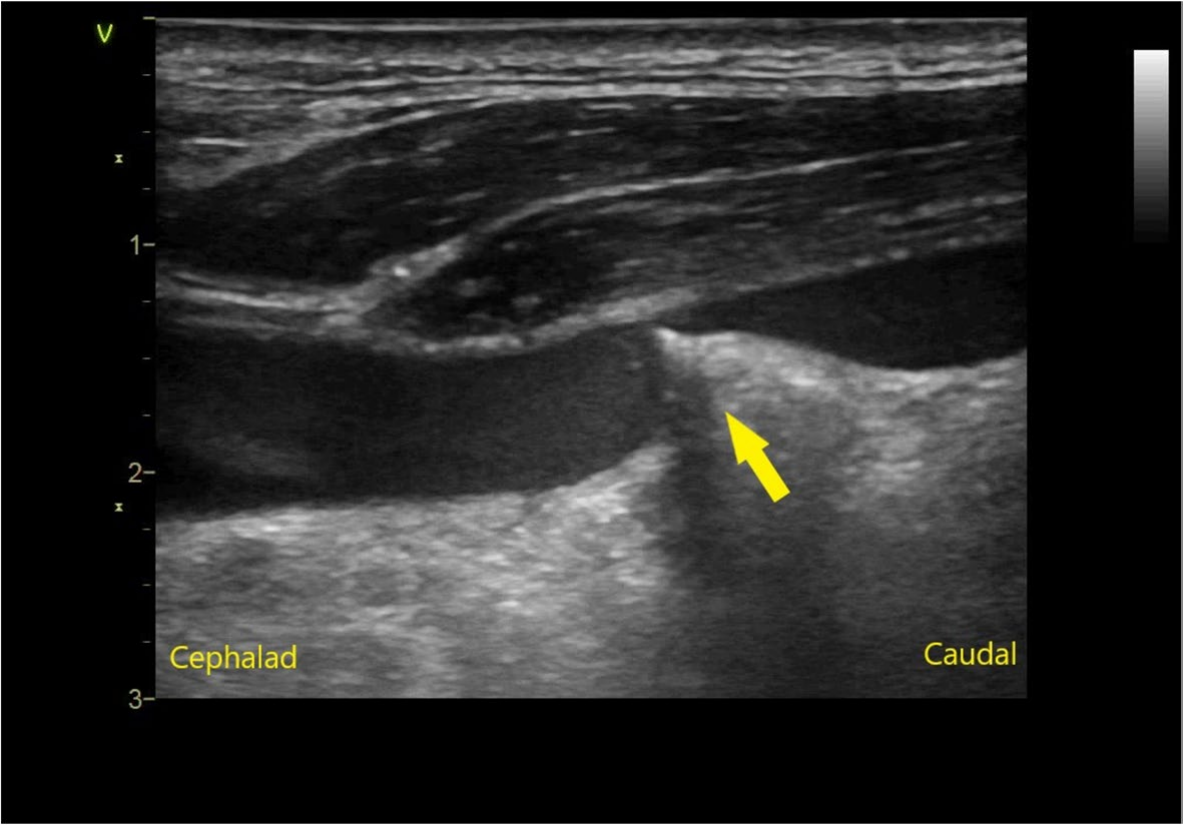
Ultrasound image of the left internal jugular vein in the longitudinal view. The posterior wall of the left internal jugular vein (arrow) is pulled ventrally by the guidewire which removal is attempted
